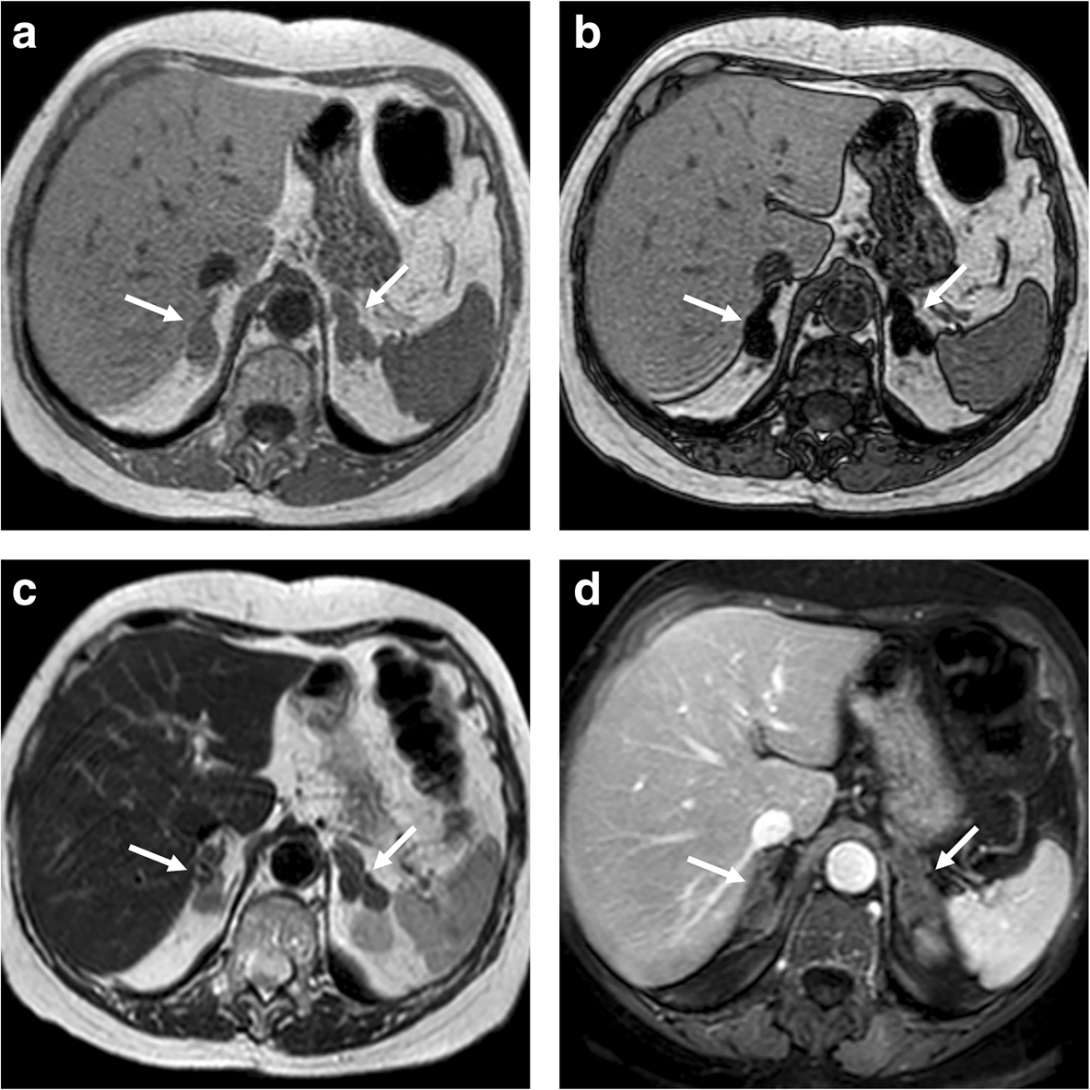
Bilateral adrenal hyperplasia in a 75-year-old woman. Axial T1-weighted in-phase (a) and out-of-phase gradient recalled echo (c), T2-weighted fast spin echo (c), and three dimensional fat-suppressed T1-weighted gradient recalled echo image in portal phase after contrast injection (d) show the nodular enlargement of both adrenal glands (arrows) with loss of signal on out-of-phase (b)
